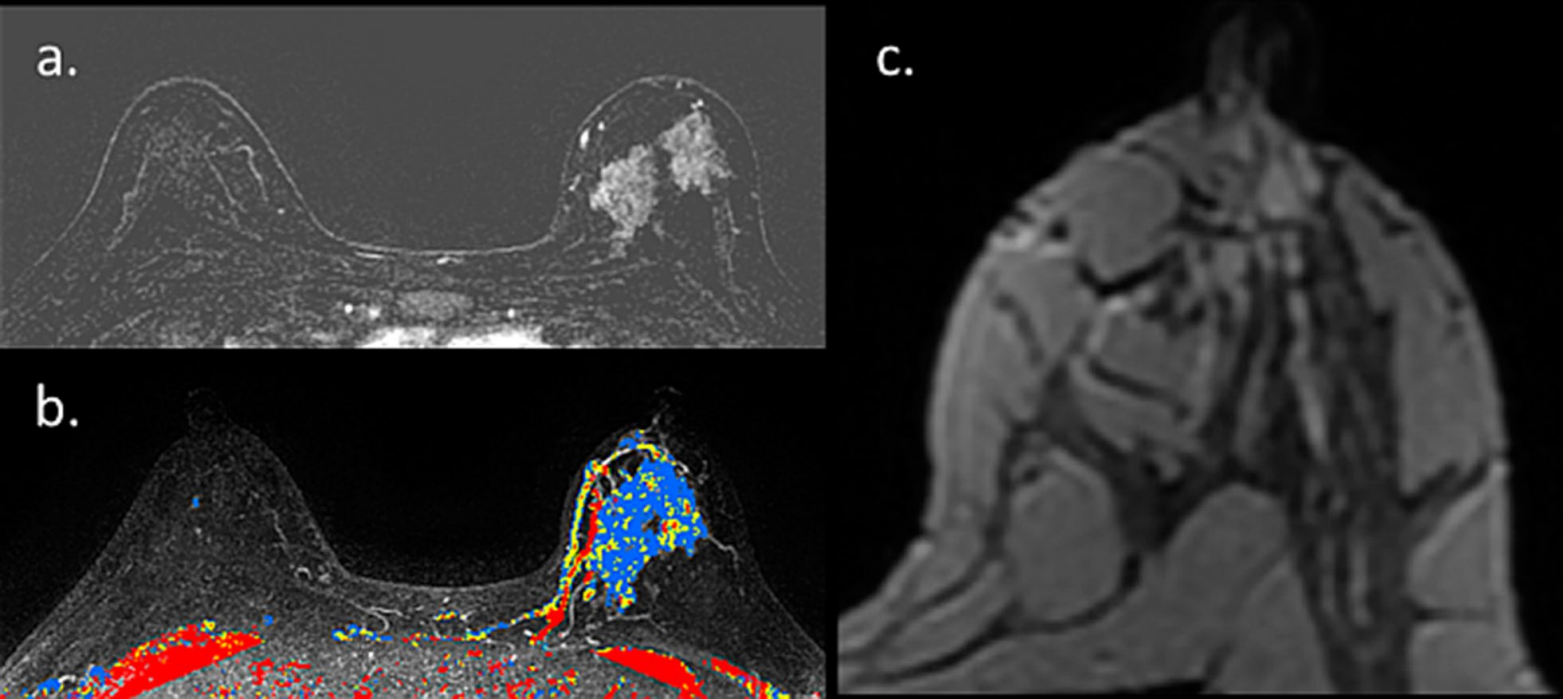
MRI of breast cancer diagnosed post-weaning. Pre-operative MR images of 42-year-old patient with DCIS, newly diagnosed to months after cessation of breastfeeding are presented. Subtraction DCE (a) and CAD (b) reveal large nonmass enhancing lesion in the left breast, on top of minimal BPE. Non-fat surpassed T2-weighted image at the height of the nipple reveal high signal ducts in the sub-areolar region (c), representing the transformation of the breast from lactation to involution
